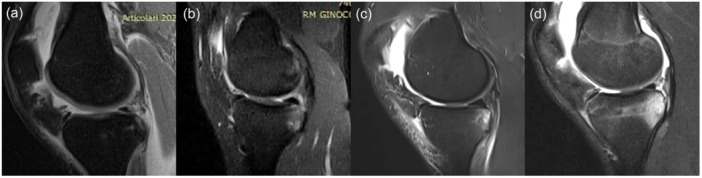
Classification of bone bruising into four grades for medial tibial plateau bone bruising on magnetic resonance imaging according to the ICRS scale: a, absent; b, minimal; c, moderate; and d, severe. ICRS, International Cartilage Repair Society.
